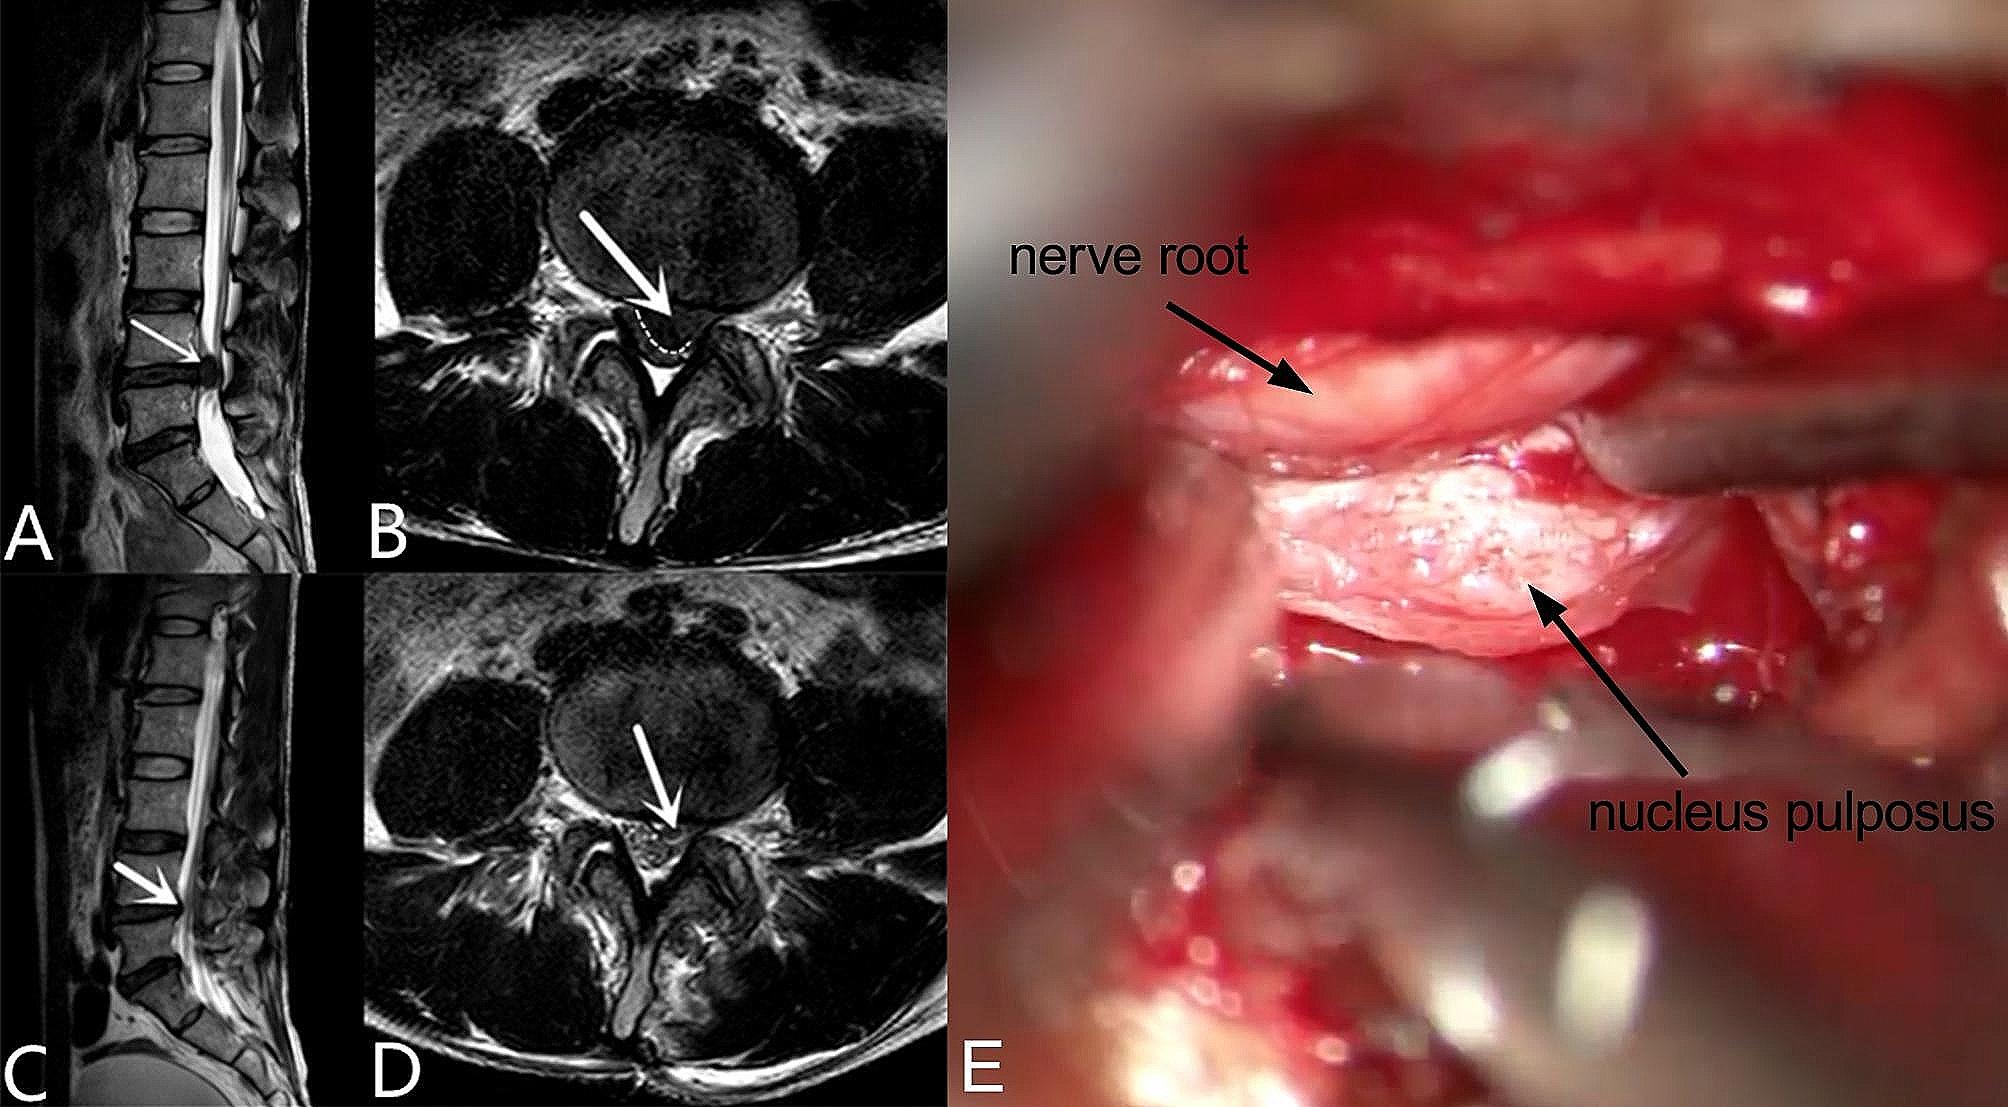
Single segment LDH observed on MRI and during surgery. A-B, massive LDH on MRI; C-D, relative small LDH; E, massive disc prolapse observed during surgery and managed with discectomy
